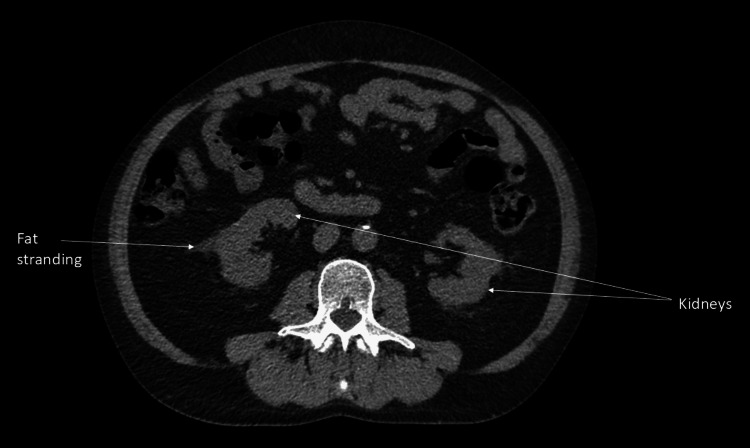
Computed tomography scan of the kidney, ureter, and bladder. Axial view of a non-contrast computed tomography scan of the abdomen demonstrating both kidneys. There are hyper-densities around the kidneys, as highlighted by the arrow, which are due to inflammation of the fat surrounding the kidneys.
